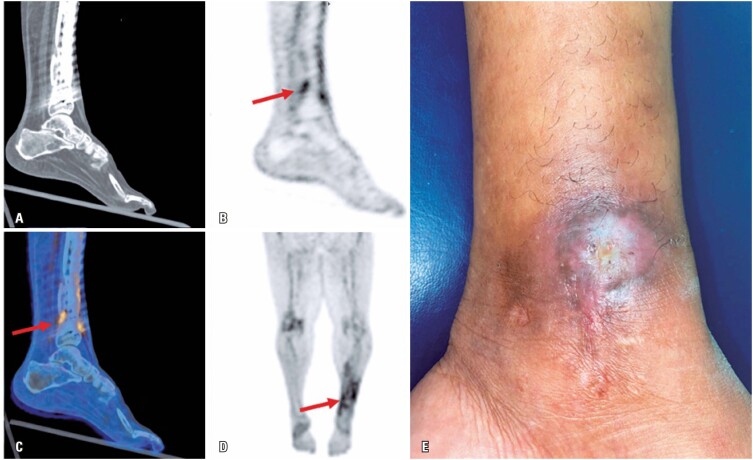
Computed tomography (A), PET (B) and fusion (C) images in sagittal plane; and image of maximum intensity projection of the tibia region (D); the red arrows indicate the ^68^Ga-UBI uptake in infection foci, and image of injury in the surgical scar on the tibia's distal region (E)
